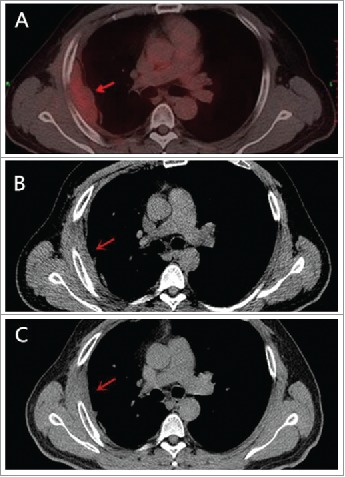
Tumor remarkable shrinkage was confirmed. PET-CT before the commencement of celecoxib on April 2014 A. CT scan on November 2015 B. and on October 2016 C. showed that tumor was smaller after using celecoxib.
